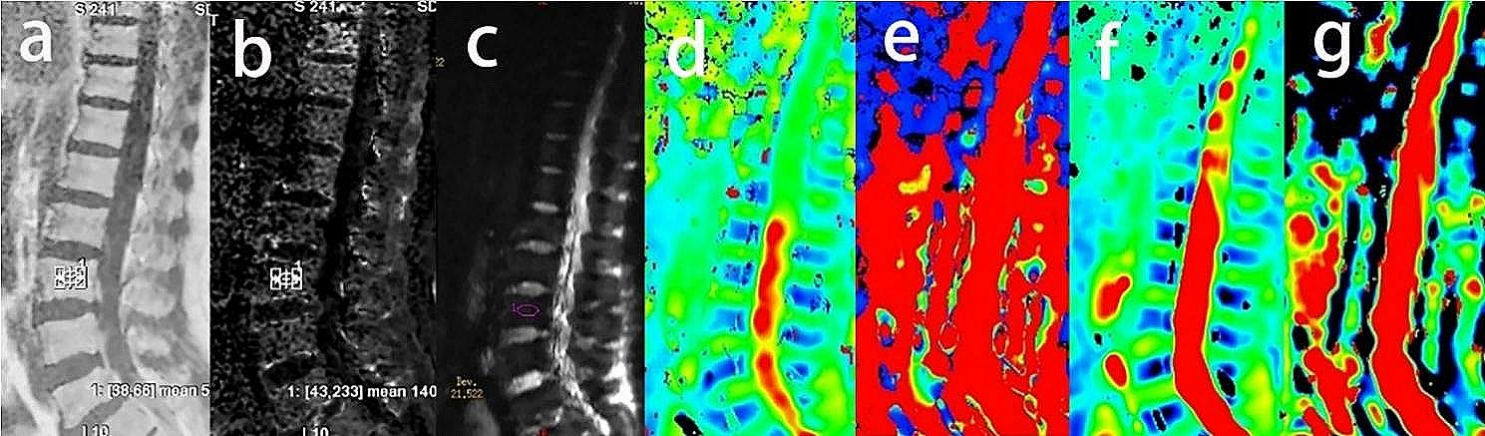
Typical image from a healthy participant without osteopenia or osteoporosis. a-g show the FF, R2*, DWI, D, D*, f, and DDC images of the lumbar spine, respectively. The circle on the vertebral body represents the measurement range. The results of d-g were shown in the left side of these original images through measuring on image c
